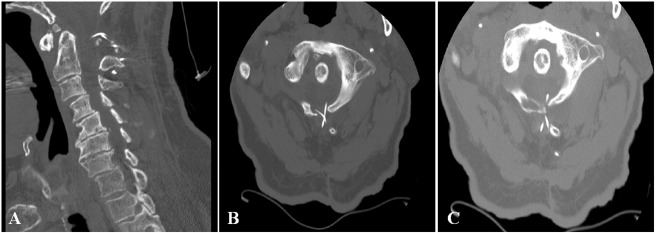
Preoperative Cervical CT Scan Cervical computed tomographic (CT) scan without contrast demonstrating sublaminar wiring in (A) sagittal and (B, C) axial views. On inspection, it appears as if the upper cervical wiring has encroached into the spinal canal with concern for dural and cord penetration.
